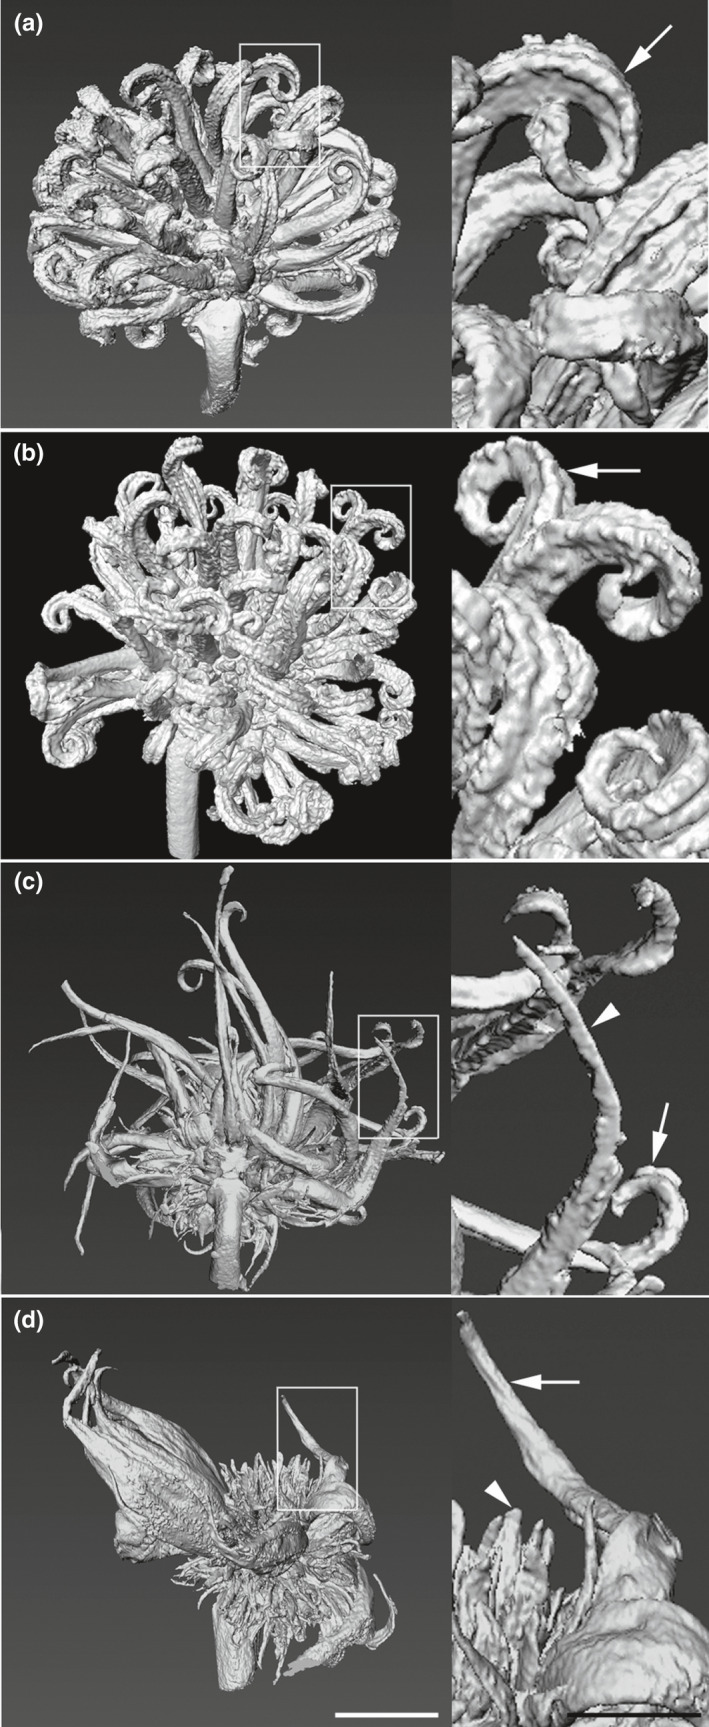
Three‐dimensional surface rendering of wild‐type and altered flowers from micro‐CT scanned images. (a) Wild‐type control female flower (CTR tree J). Higher magnification image (right) shows rough‐textured stigmas (arrow). (b) Wild‐type RNAi‐*AG* female flower event P78‐1. Higher magnification image (right) highlights rough‐textured stigmas (arrow). (c) Modified female RNAi‐*AG* event P134‐1 flower. Higher image (right) shows the diversity of long projections with some (arrowhead) lacking apparent stigmas and others with poorly developed stigmas (arrow). (d) Modified female RNAi‐*AG* event J94‐4 flower bud. Higher image shows a long projection lacking a stigma (arrow) and the presence of flat floral organs (arrowhead). Flowers were collected May of 2018, left scale bar is 1,000 μm, right scale bar is 500 μm
